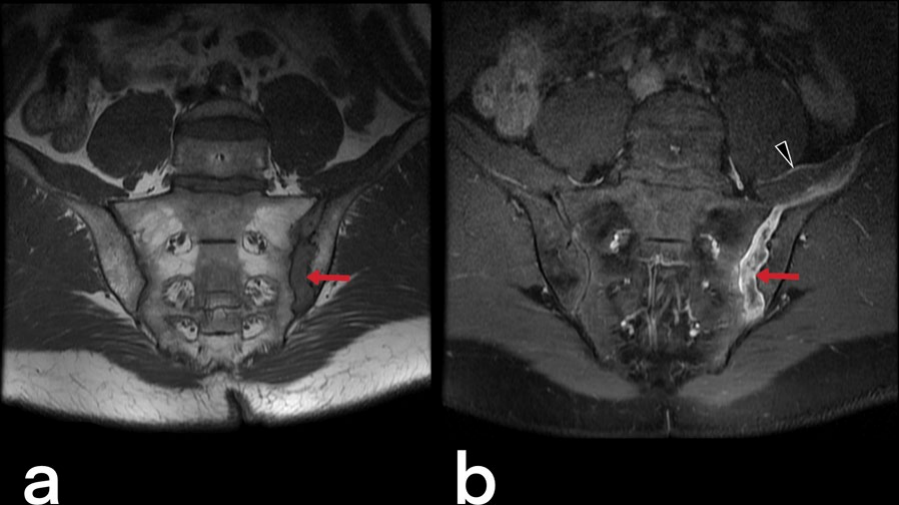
MR images of a 43-year-old male. Pre-contrast T1 weighted (a) and post-contrast (b) T1 weighted images demonstrate contrast enhancement in the synovium of the left sacroiliac joint in keeping with acute sacroiliitis (arrows). Arrowhead shows an abscess formation in the left iliac muscle that enhances peripherally after contrast injection
